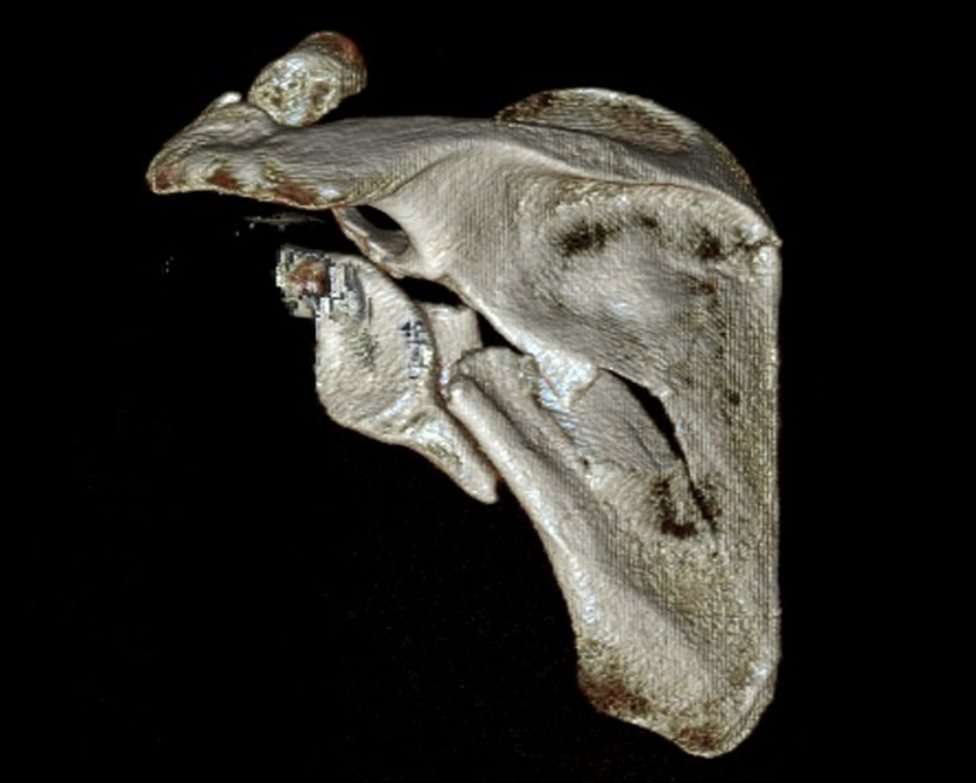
Left scapular fracture – 3D CT reconstruction.
